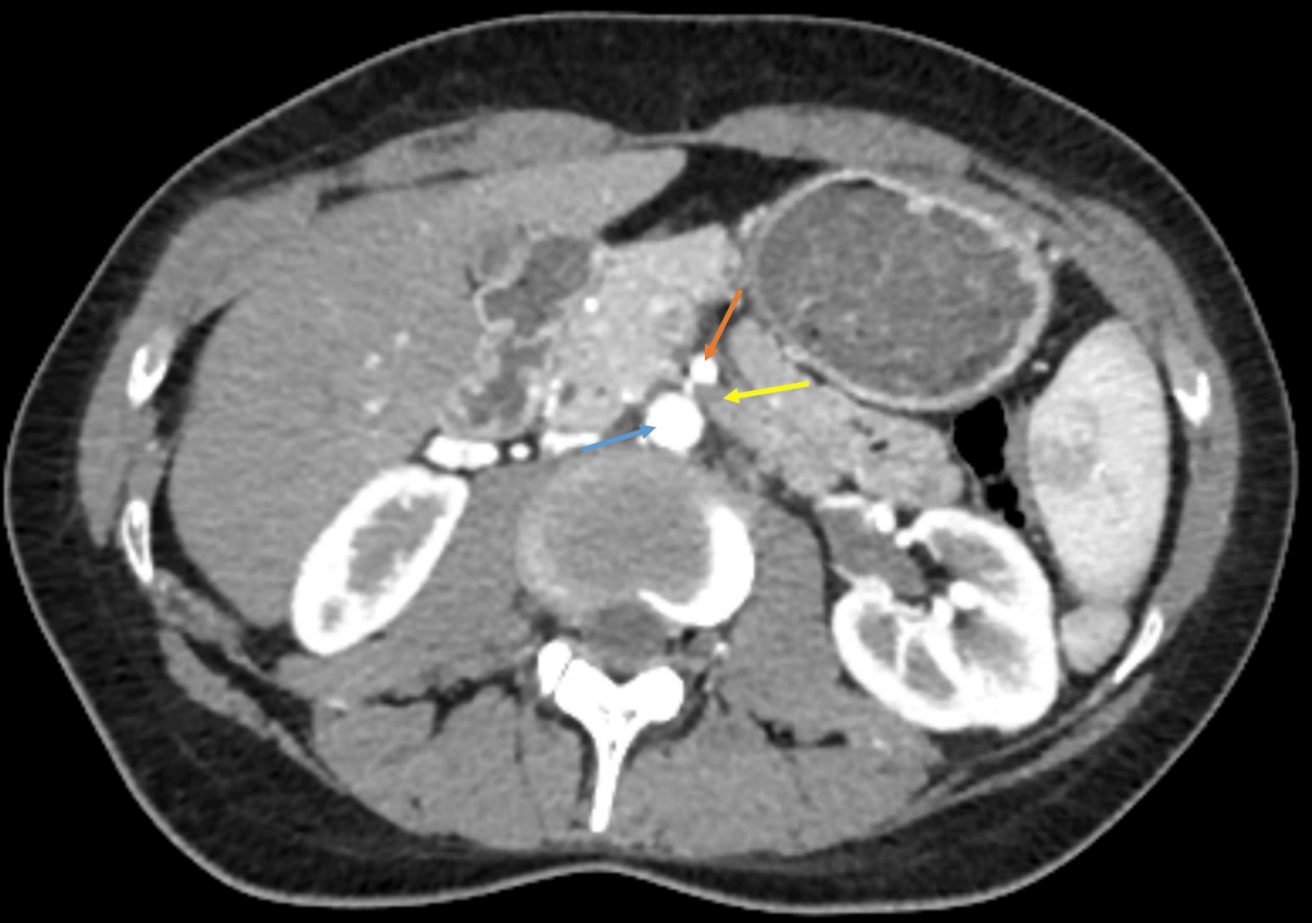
Axial CT image showing duodenal compression (yellow arrow) by the SMA (orange arrow) and the aorta (blue arrow) CT: Computed Tomography; SMA: Superior Mesenteric Artery
